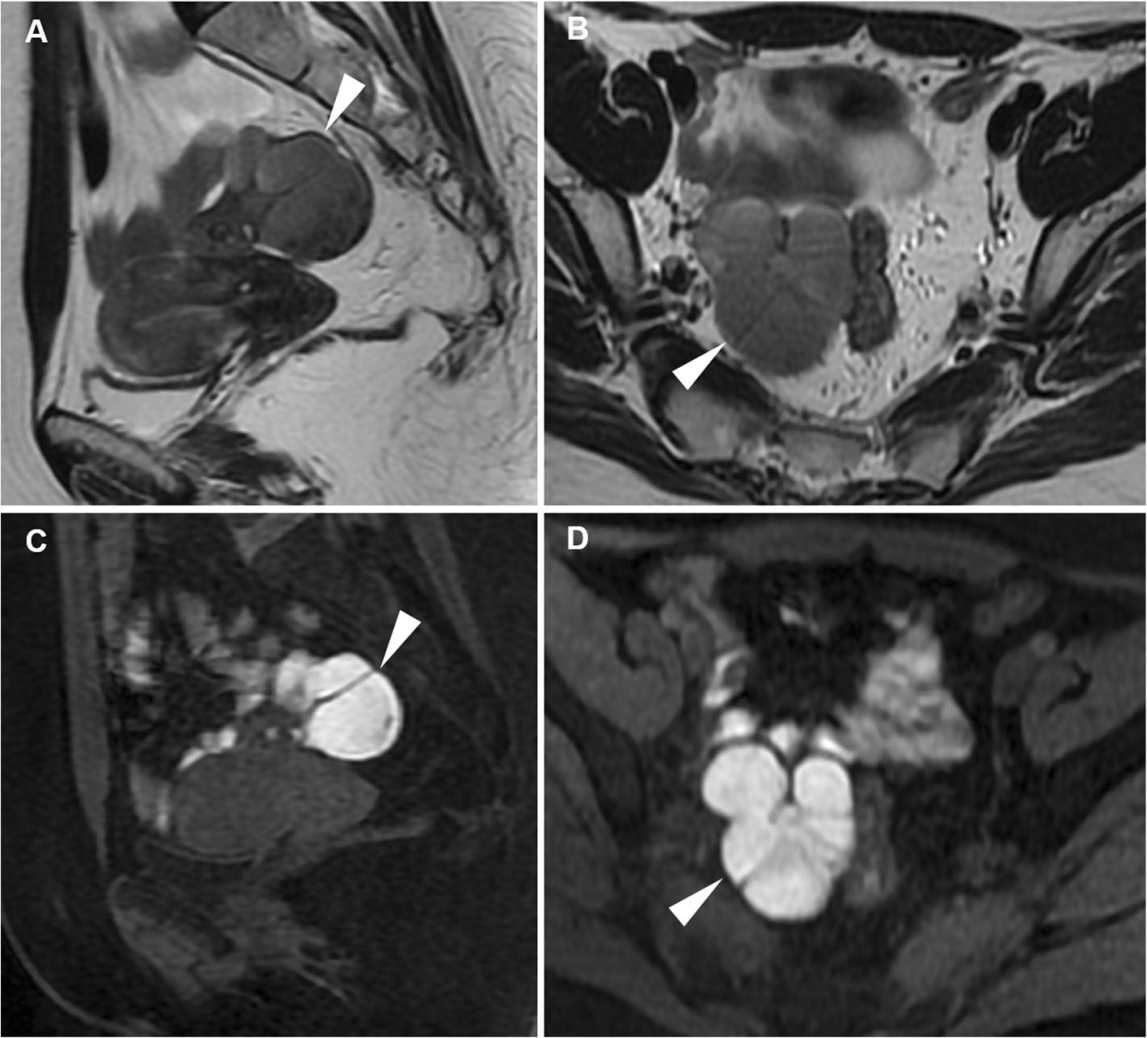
Haematosalpinx in a 34-year-old woman with endometriosis presenting with dysmenorrhea and pelvic pain. Sagittal (a) and oblique-coronal (b) T2-weighted images show a tortuous structure with homogeneously hypointense content in right adnexa (arrowheads). On sagittal (c) and oblique-coronal (d) fat-suppressed T1-weighted images the same structure (arrowheads) shows high signal intensity consistent with haemorrhagic content
